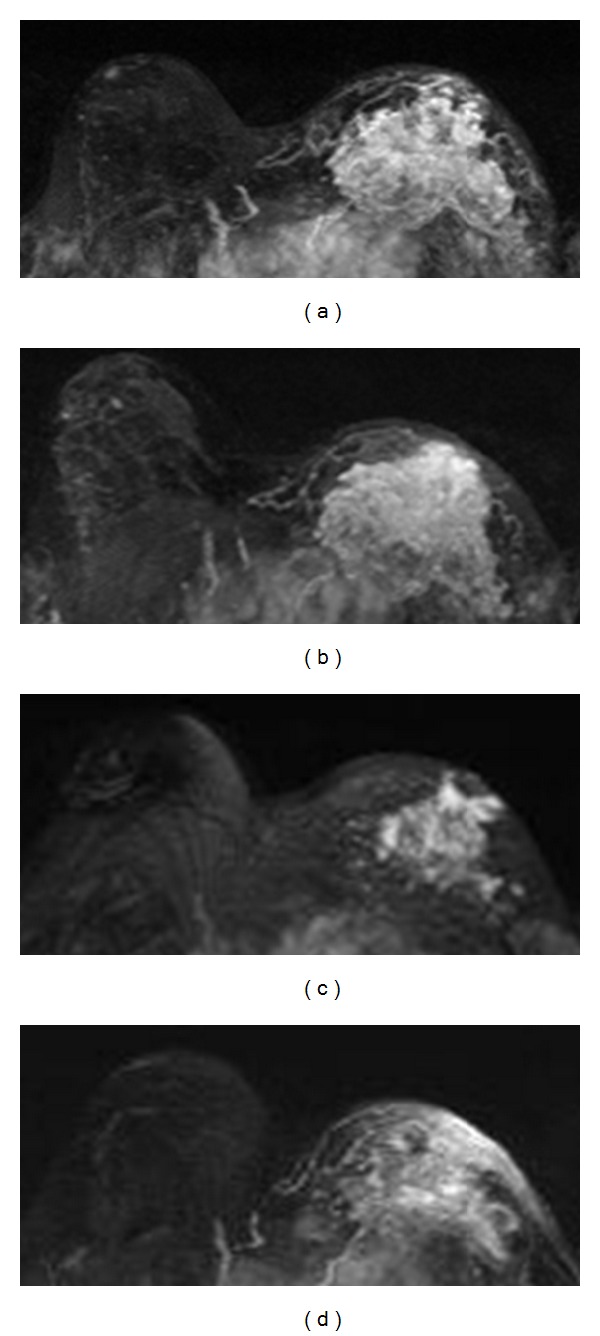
A 29-year-old patient with non-mass-like enhancement lesion in the left breast. From (a) to (d), the maximum intensity projection (MIP) images of pretreatment, F/U-1, F/U-2, and F/U-3 MRI are shown. The extent of tumor size is 8.2 cm before treatment, remains about the same at 8.0 cm in F/U-1, shrinks down to 4.5 cm in F/U-2, and progresses again to 6.2 cm in F/U-3. The choline measured by MRS shows [tCho] = 0.77 ± 0.11 mmol/kg before treatment, which decreases to 0.20 mmol/kg in F/U-1, and then increases to 1.01 mmol/kg in F/U-2, and further increases to 1.70 mmol/kg in F/U-3. The transient decrease of tCho in F/U-1 precedes the size reduction observed later in F/U-2. And then the increase of tCho in F/U-2 indicates treatment failure, and the tumor grows larger in F/U-3.
